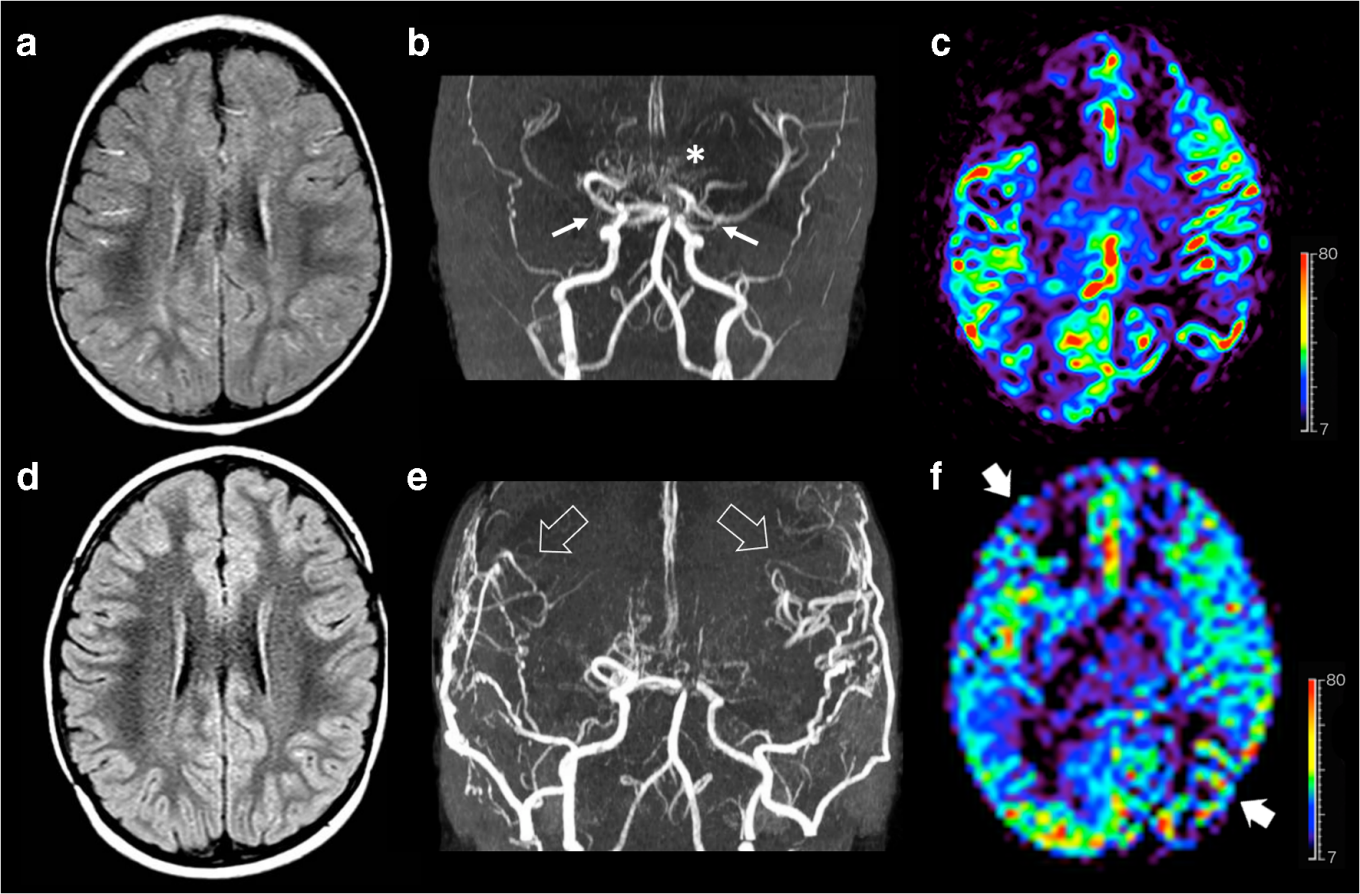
Brain MRI studies with MRA and arterial spin labeling (ASL) perfusion acquired before (a–c) and 1 year after surgery (d–f). a Axial FLAIR image reveals linear high signal intensity along the cortical sulci in both cerebral hemispheres, in keeping with diffuse prominent leptomeningeal collaterals. b Arterial MRA shows bilateral moyamoya vasculopathy with stenosis of distal portion of both internal carotid arteries (arrows), severe stenosis of right middle cerebral artery, posterior circulation involvement, and basal moyamoya collateralization (asterisk). c ASL perfusion map reveals hypoperfusion of both middle cerebral artery territories, in particular of the right frontal lobe and left parietal lobe. d Axial FLAIR image after surgery demonstrates resolution of the leptomeningeal collaterals. e Postoperative MRA demonstrate development of pial collateralization in the sites of surgical revascularization (arrowheads). f ASL perfusion map unravels marked improvement of the CBF in the middle cerebral artery territories after surgery (thick arrows). Color bar indicates ml/min/100 g for ASL-CBF.
